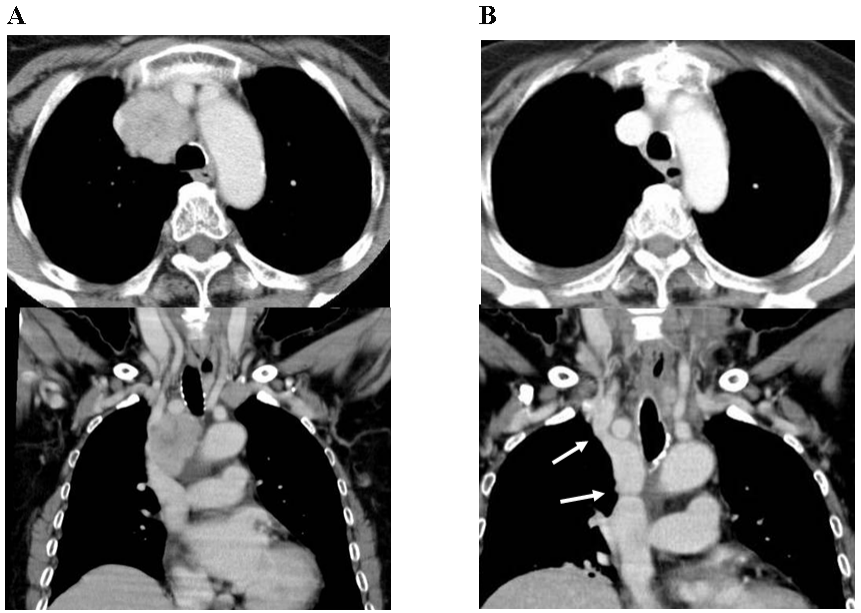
A: Enhanced computed tomography (CT) reveals stenosis of the superior vena cava (SVC) due to invasion of the mediastinum lymph node metastasis (LNM). B: Postoperative CT scan shows the patency of the venous pathway after resection and reconstruction with the autograft (left brachiocephalic vein). Two arrows indicate the sites of distal and proximal anastomosis.
